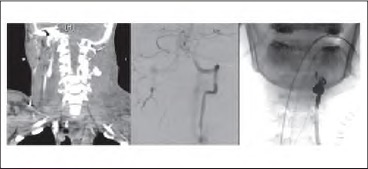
Computed tomography angiography and angiography demonstrating arteriovenous fistula of left vestibular artery and extensive endovascular coiling
